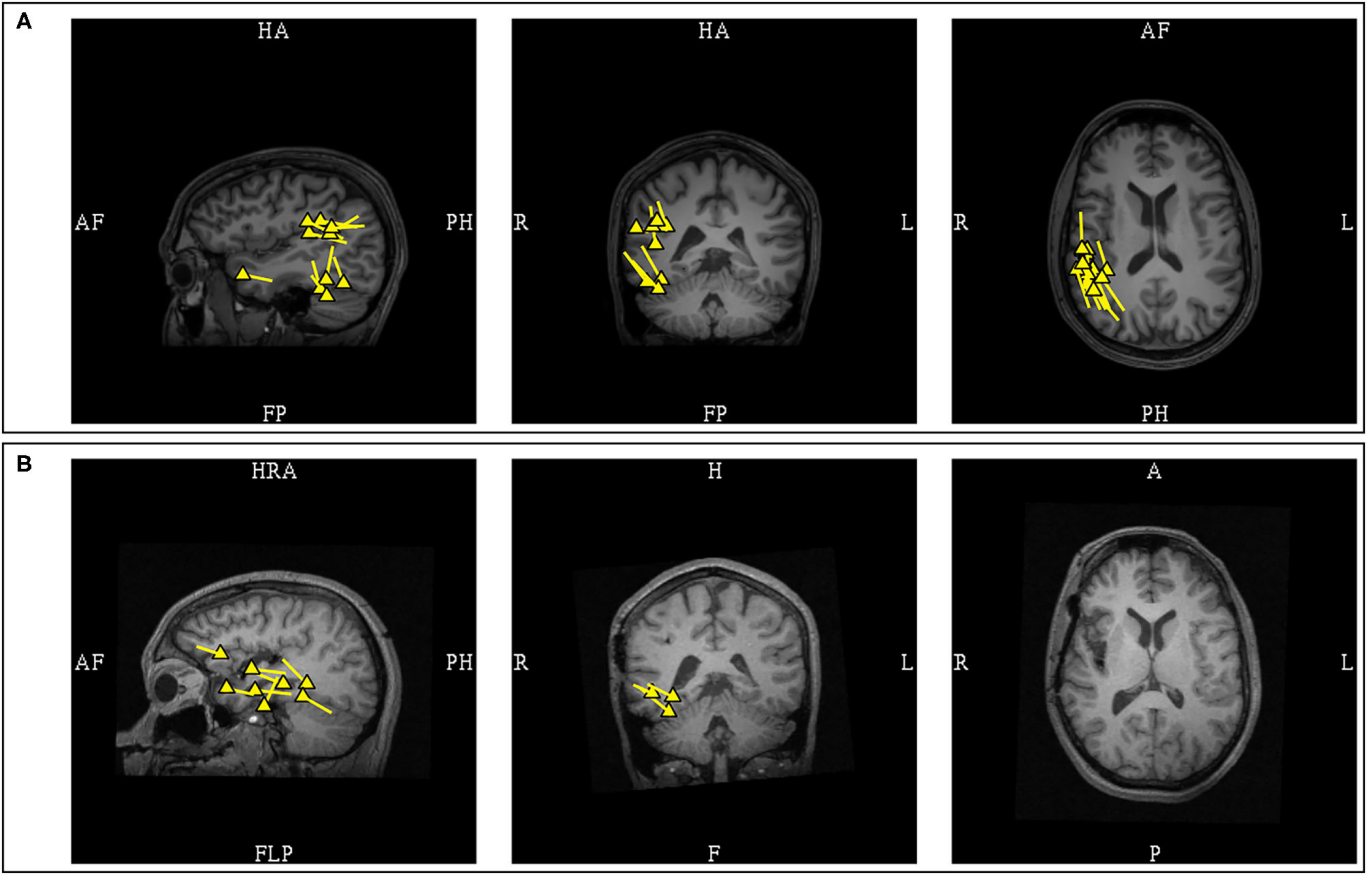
MEG-MRI coregistration summary in a patient with a previous right anterior temporal laser ablation who underwent posterior insular resection. (A) Pre-operative MEG showed multifocal clusters over the right supramarginal, posterior basal temporal, and anterior temporal regions. The supramarginal cluster in this patient was pathologic due to presence of an epileptiform correlate. Invasive EEG recording confirmed interictal spiking concordant with MEG. (B) Post-operative MEG after the posterior insular resection showed disappearance of the supramarginal cluster, but the temporal dipoles remained.
